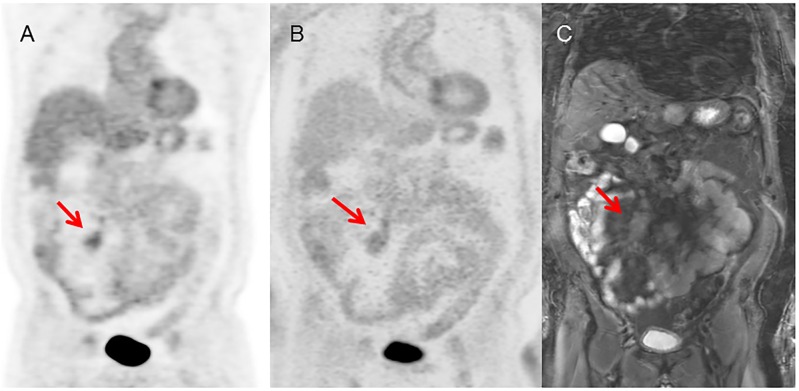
Concordant lesion, identified prospectively on both modalities. 66 year-old male with neuroendocrine tumor who underwent PET/CT for initial staging. (A) PET image from PET/CT, (B) PET image from PET/MRI, (C) MRI (STIR) image. Both PET/CT and PET/MRI could identify the mesenteric lesion (arrow).
